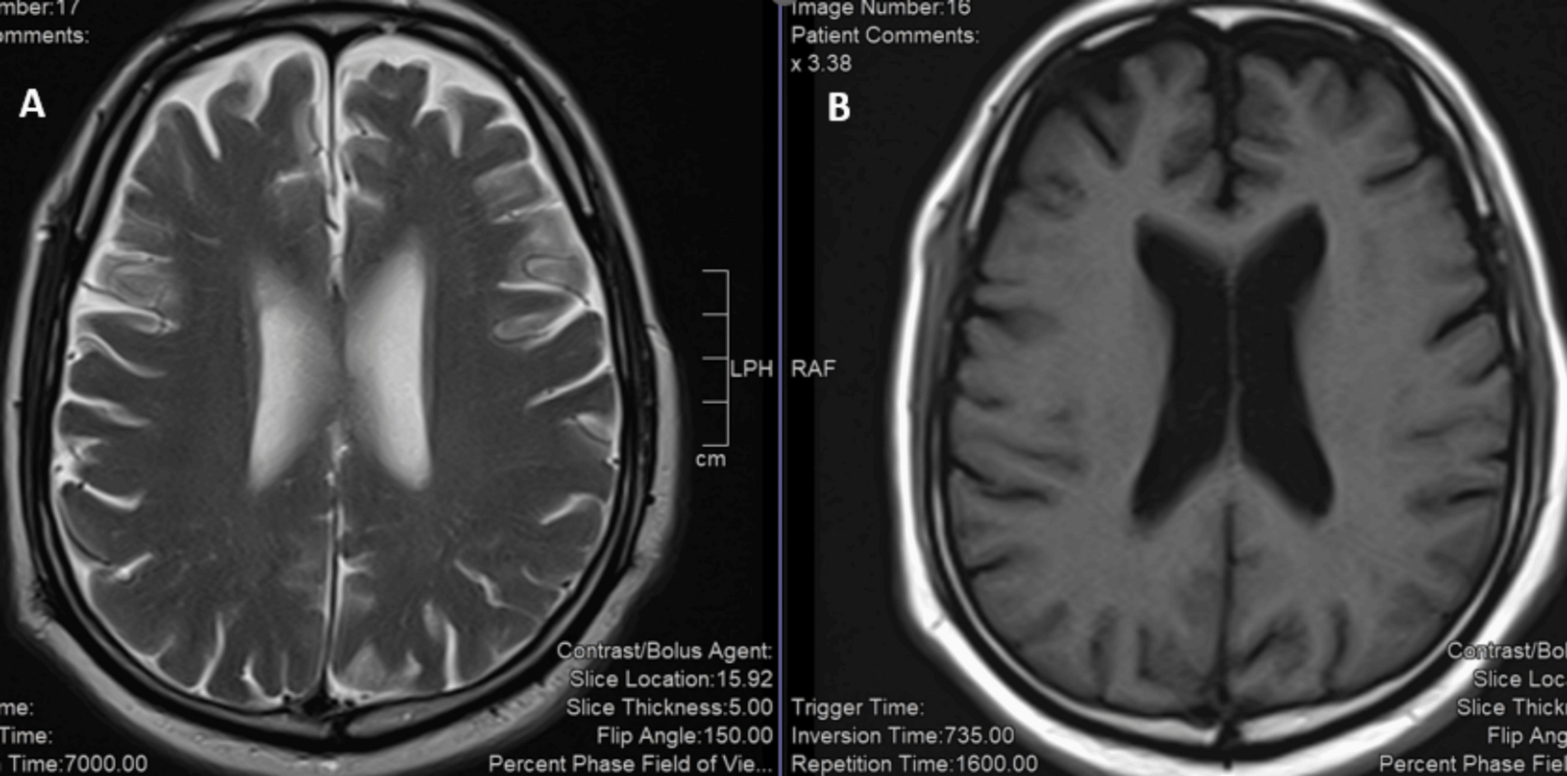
(A) T2-weighted and (B) T1-weighted imaging shows normal brain structures and signal intensities.
